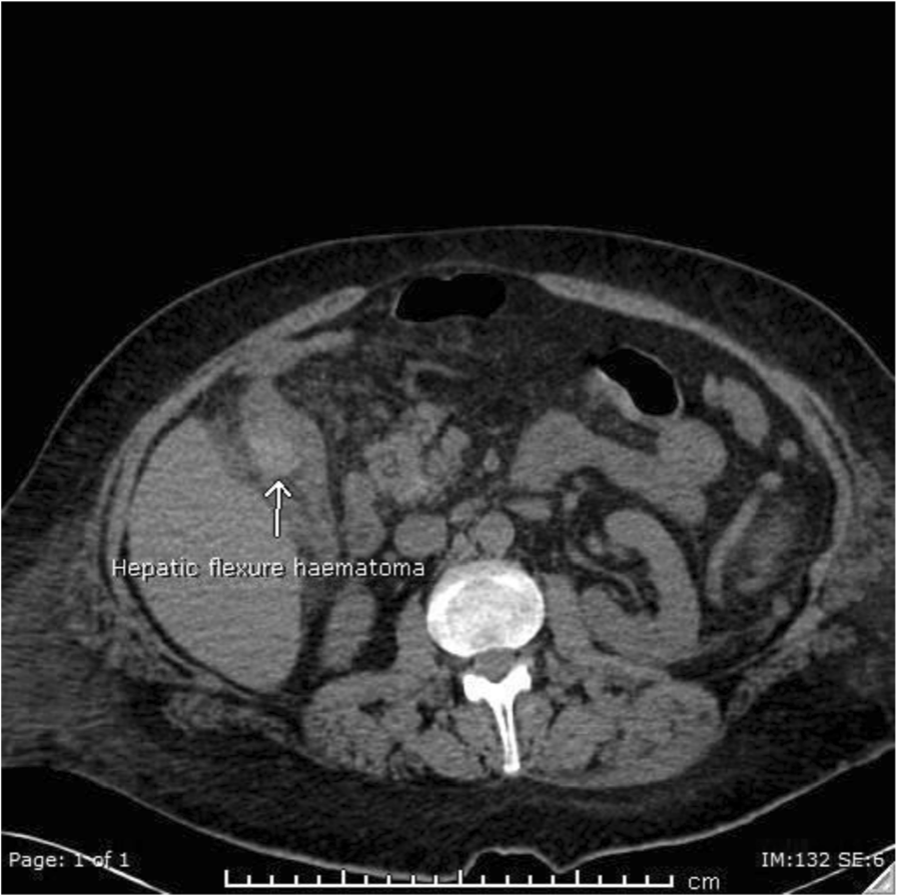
Pre-contrast CT. Dense fluid within the hepatic flexure
